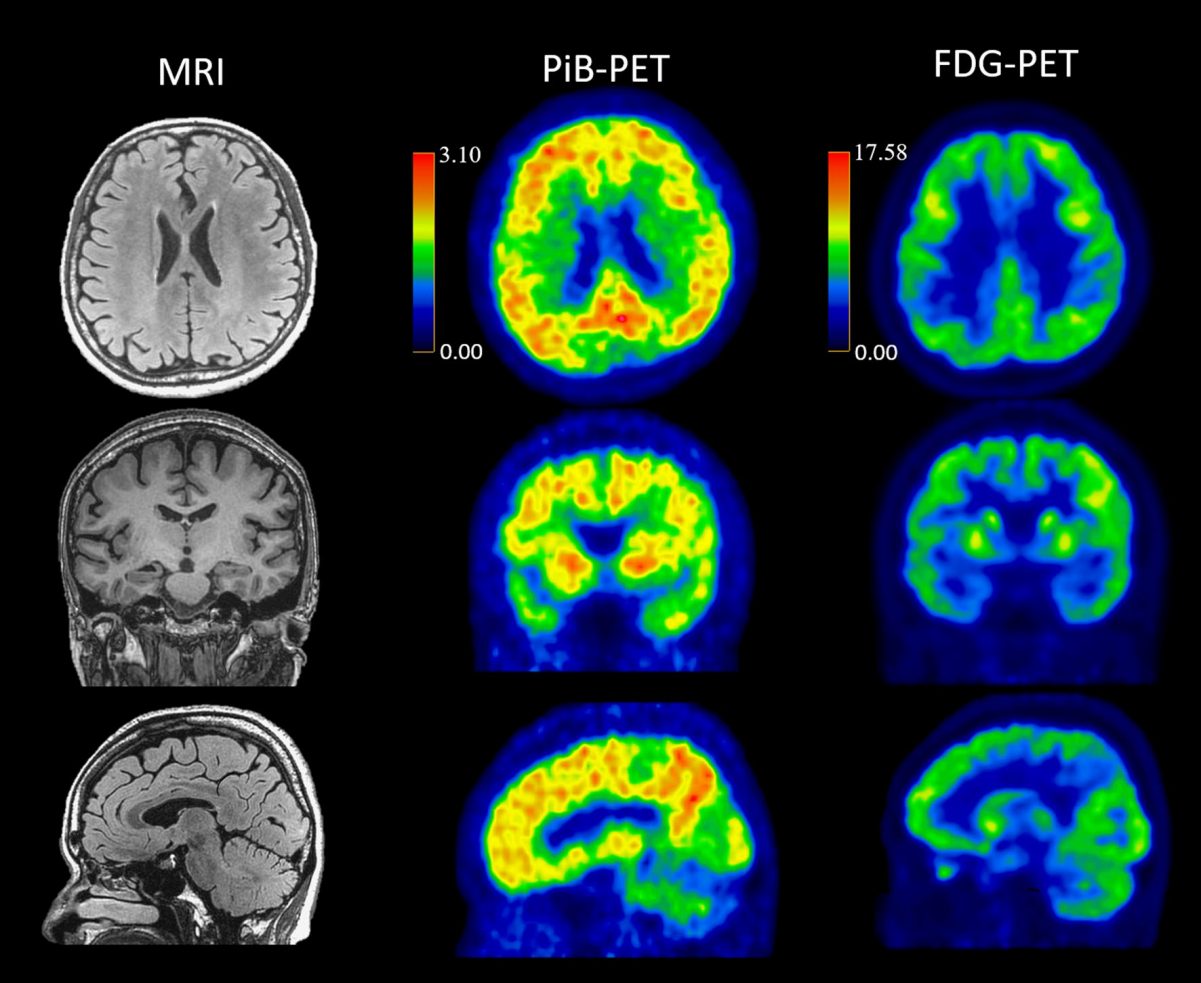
Brain imaging of a patient with *PSEN1* p.His131Arg and age at onset of 49 years. Brain MRI at age 61 and PiB-PET and FDG-PET at age 62 were showed. MRI showed mild medial temporal atrophy, mild parietal atrophy and minimal white matter hyperintensity; PiB-PET demonstrated extensive cortical and striatal amyloid deposition; FDG-PET showed decreased uptake in precuneus and bilateral temporo-parietal region.
